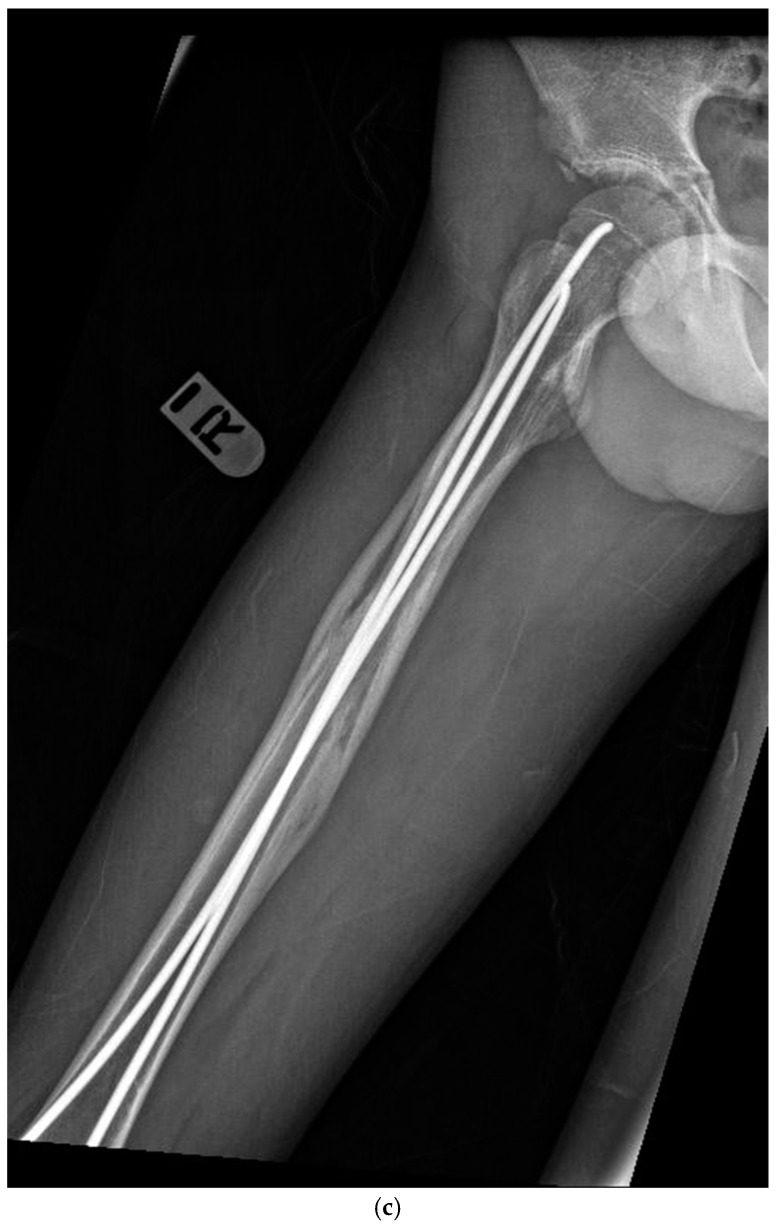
A 12-year-old male with a butterfly subtrochanteric fracture. (a) Immediate postoperative radiograph. (b,c) Twelve-week postoperative radiographs, anteroposterior and lateral views.
